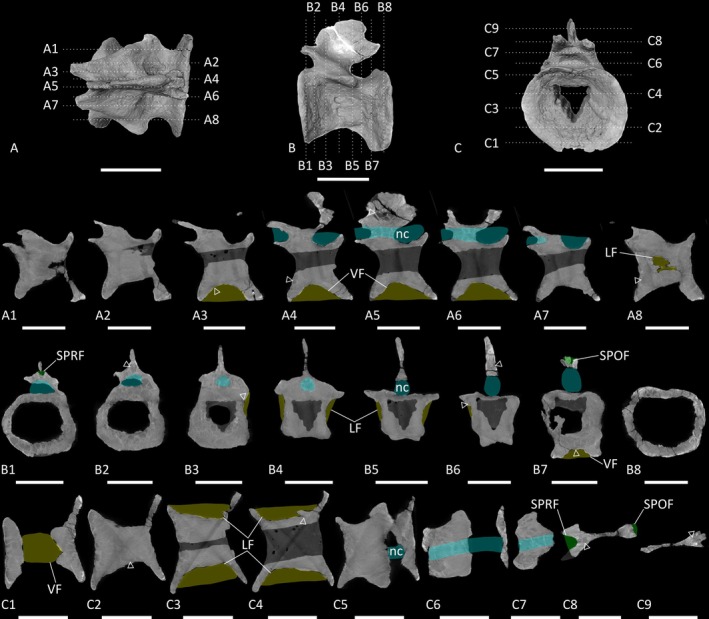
CT scan of *Giraffatitan brancai* caudal vertebra 15 MB.R.5000.14. (A–C) 3D rendering (A) dorsal view, (B) left lateral view, (C) anterior view; (A1–A8) CT scan slices in parasagittal cross‐section, (B1–B8) CT scan slices in transverse cross‐section, (C1–C9) CT scan slices in horizontal cross‐section. LF, lateral fossa; nc, neural canal; VF, ventral fossa. Key: Blue highlight, neural canal; green highlight, neural spine fossa; yellow highlight, centrum fossa; white arrow, pneumatic bone texture. Scale bars = 100 mm.
